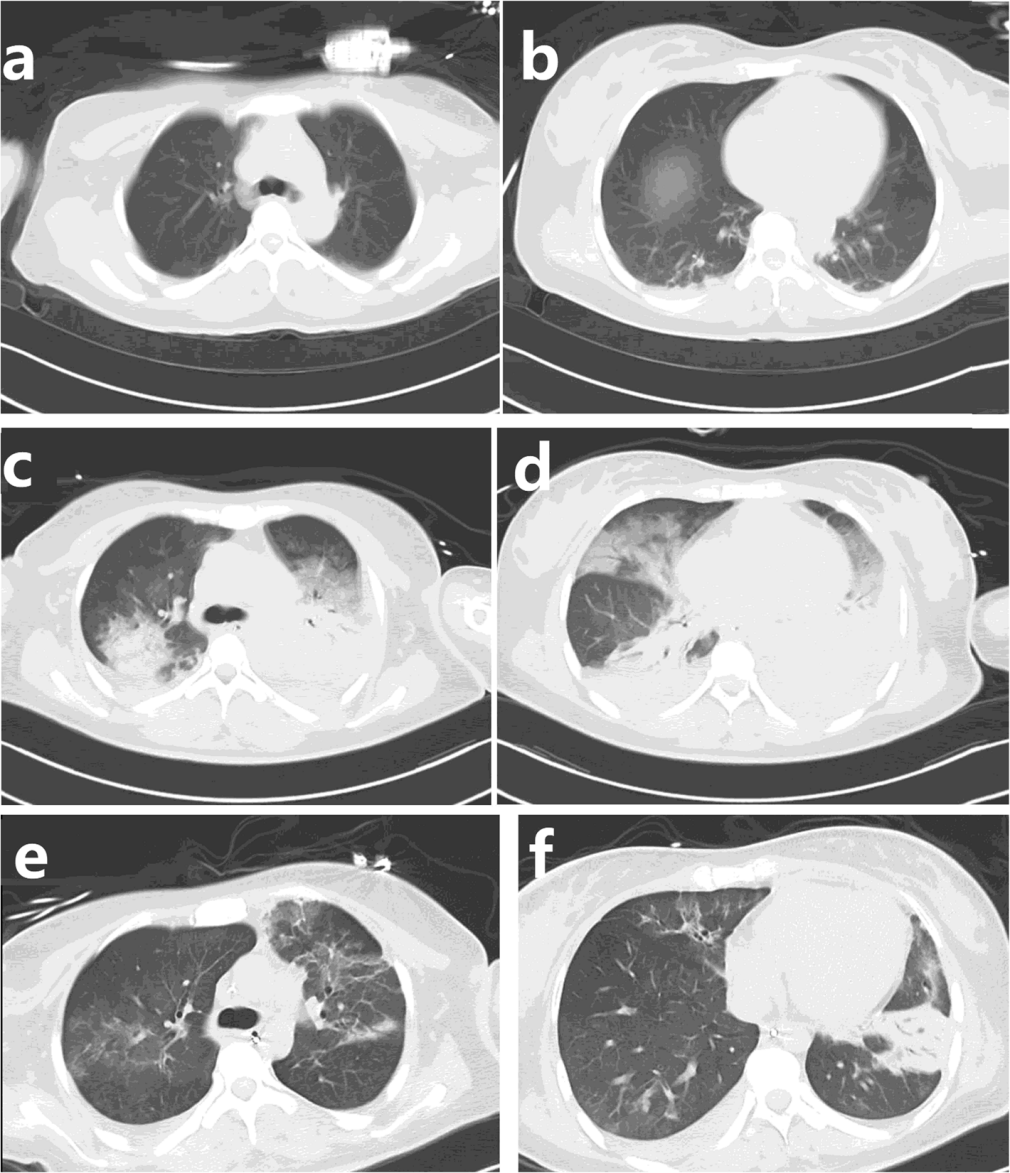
CT findings of lung: a and b The computed tomography (CT) showed mild exudation in the lower lobe of the lung which revealed a pneumonia at admission. c and d The CT on day 12 showed the involvement of the lung had progressed. e and f The CT scan on day 24 showed clearer lung field and less infiltration and atelectasis, and the pneumonia was improved
